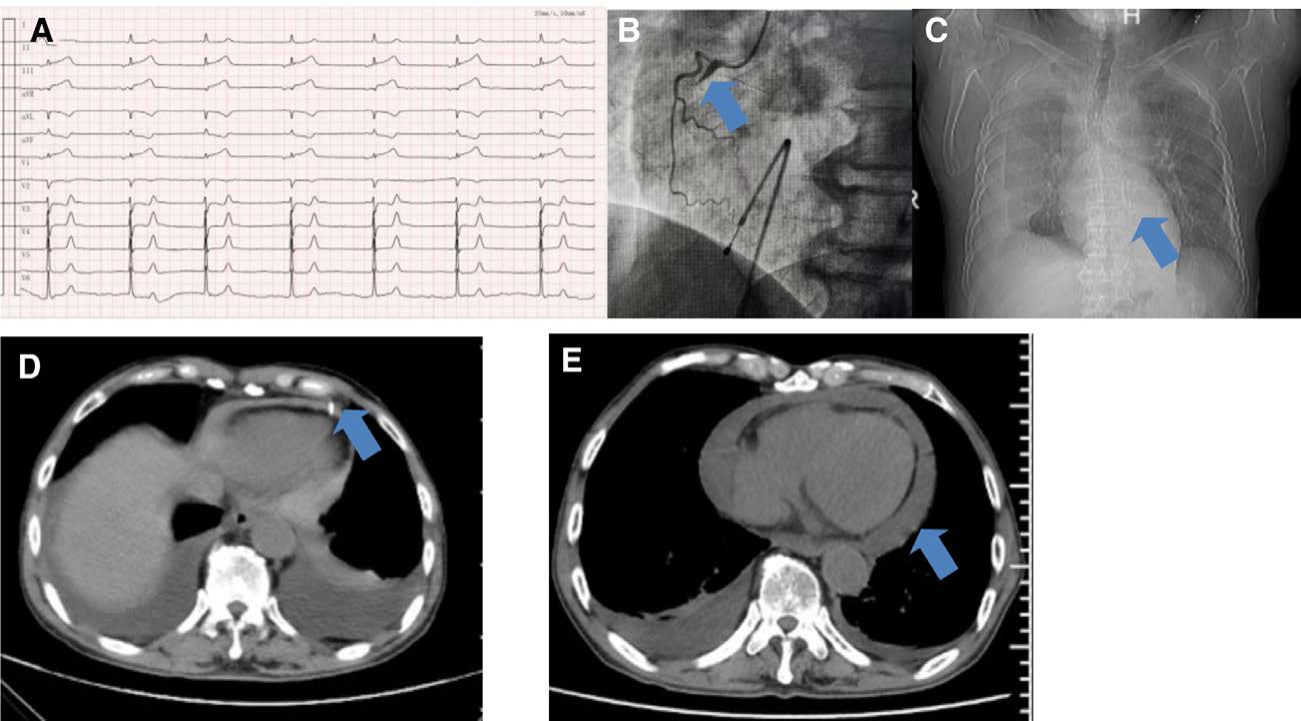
(A) Junctional rhythm (40 bpm) with ST-segment elevation in leads II, III, and aVF leads; (B) coronary angiography under temporary pacing showing complete occlusion of the proximal right coronary artery; (C and D) pericardial catheter placement. (E) Chest CT showing recurrence of pericardial effusion. CT = computed tomography.
